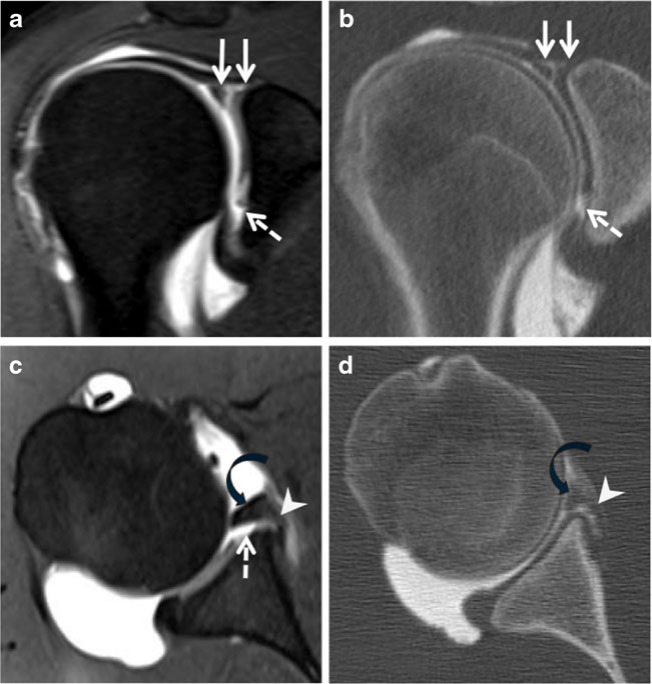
Bucket-handle SLAP tear (Type 3) with Perthes lesion in a 23-year-old male athlete with a one-month history of right shoulder pain after a fall onto outstretched arm. a Coronal and c axial fat-suppressed T1-weighted MR arthrography with correspondent b coronal and d axial CT arthrography images. Presence of a Bucket handle tear of the labro-bicipital complex extending across the bicipital attachment (*white plain arrows*) and to the anteroinferior labrum (*11*–*6 o’clock*). The anteroinferior labrum is avulsed (*black curved arrow*) with medial stripping of an intact scapular periosteum (*white arrowhead*) consistent with a Perthes lesion. There is a cartilage defect of the anteroinferior labrum (*dashed arrow*). These findings can be classified either as a SLAP III (with regard to the bucket handle tear) with Perthes lesion, or SLAP V (with regard to the anteroinferior extension of the labral tear)
